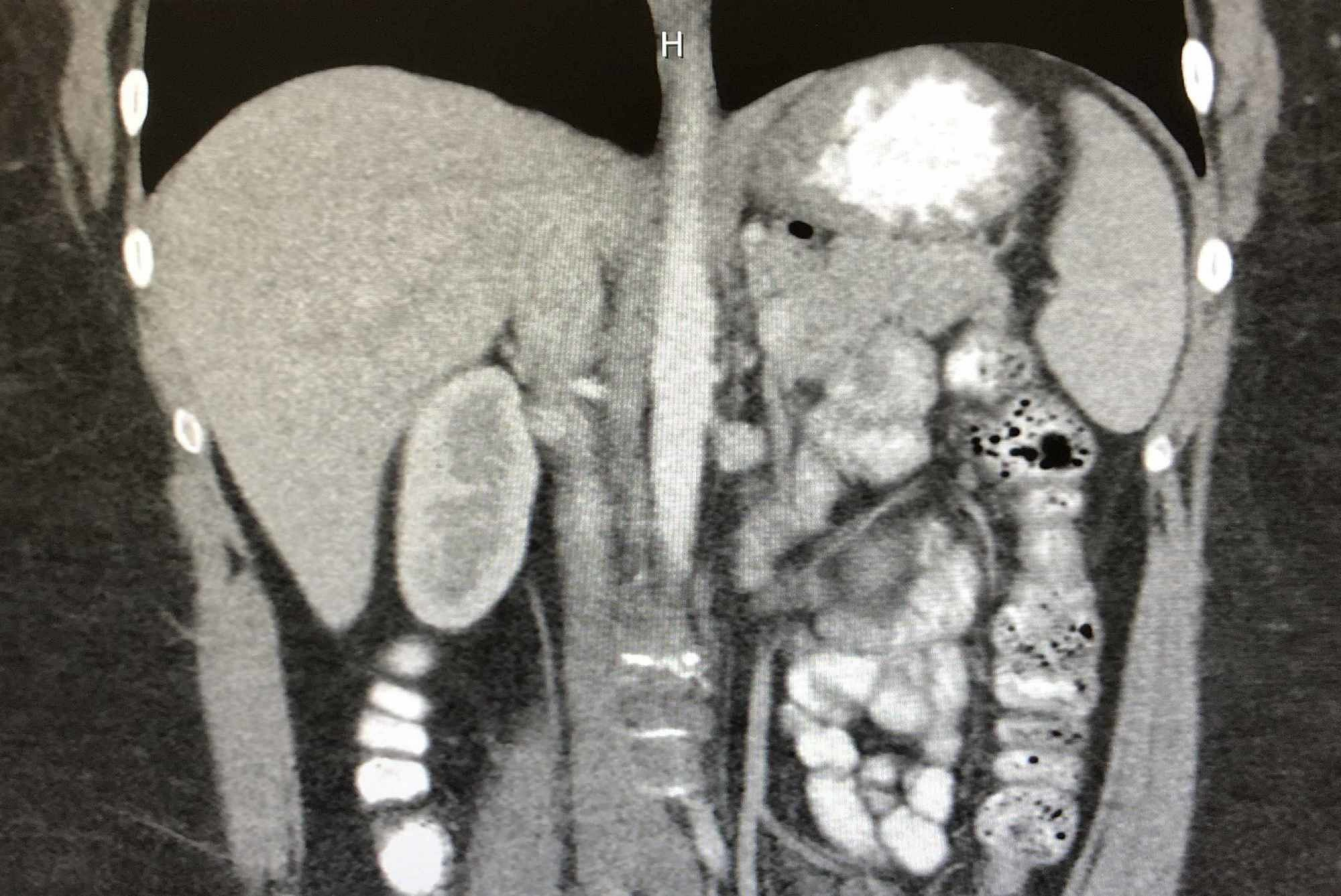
Coronal preoperative CT scan was unremarkable.
